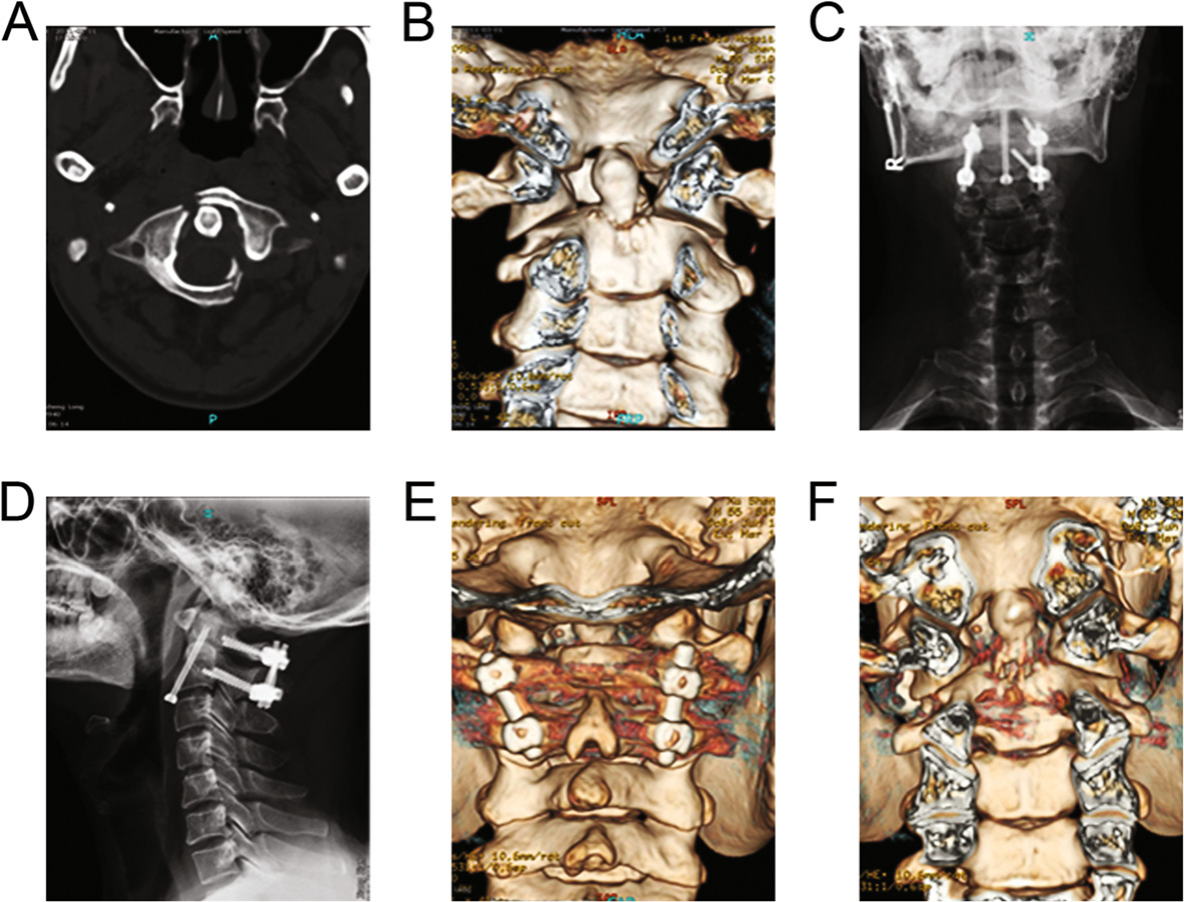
A 34-year-old male patient developed pain symptoms, limited neck movement, and numbness of his two upper extremities after traffic accidents. CT scanning (A) and 3-D CT (B) indicated fractures of the odontoid process of the axis and C1 before operation. Anteroposterior (C) and lateral (D) cervical spine X-ray scanning showed a fracture line in the odontoid anterior after anterior screw and posterior atlantoaxial pedicle screw fixation. 3-D CT showed bone fusion (E) and fracture healing (F) at 6 months after operation.
